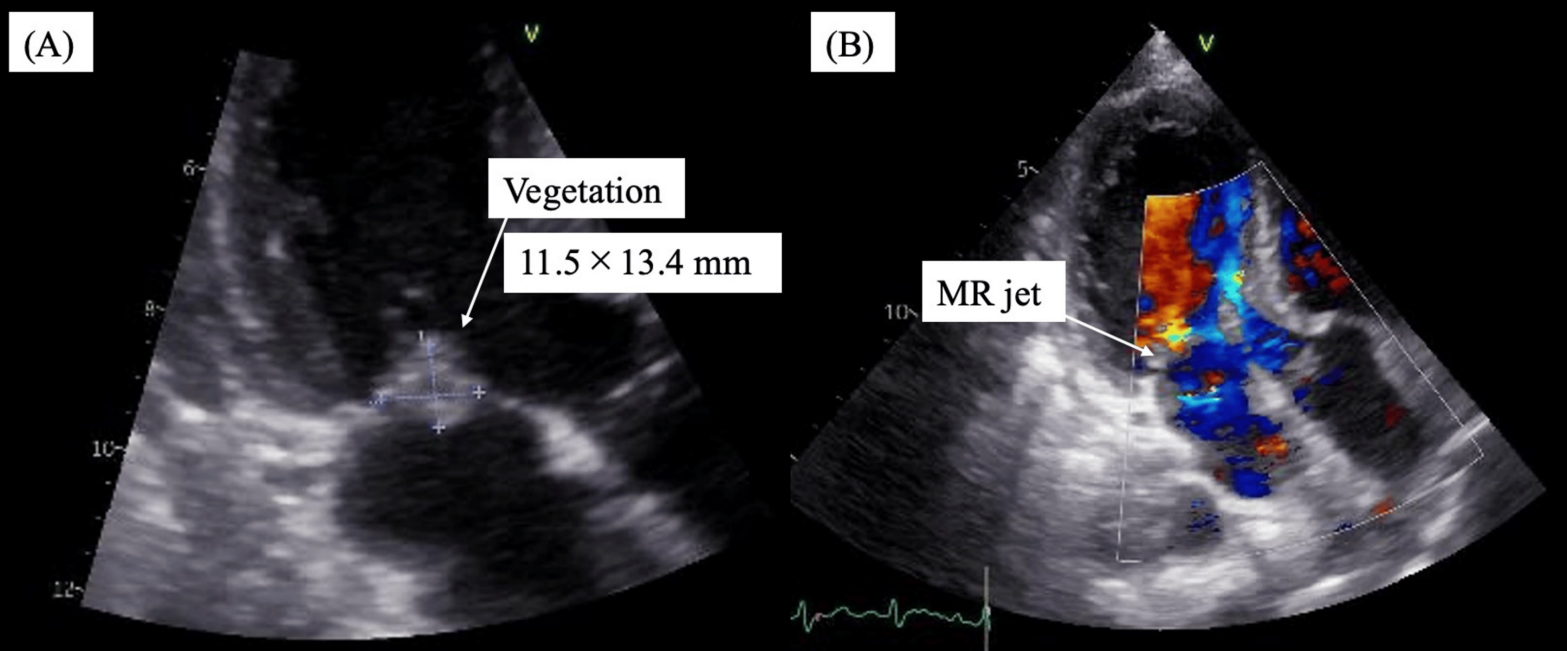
Transthoracic echocardiography on day six showing infective endocarditis (A) B-mode image showing a vegetation measuring 11.5 × 13.4 mm on the anterior mitral leaflet. (B) Color Doppler image obtained at the same imaging plane demonstrating mitral regurgitation.
